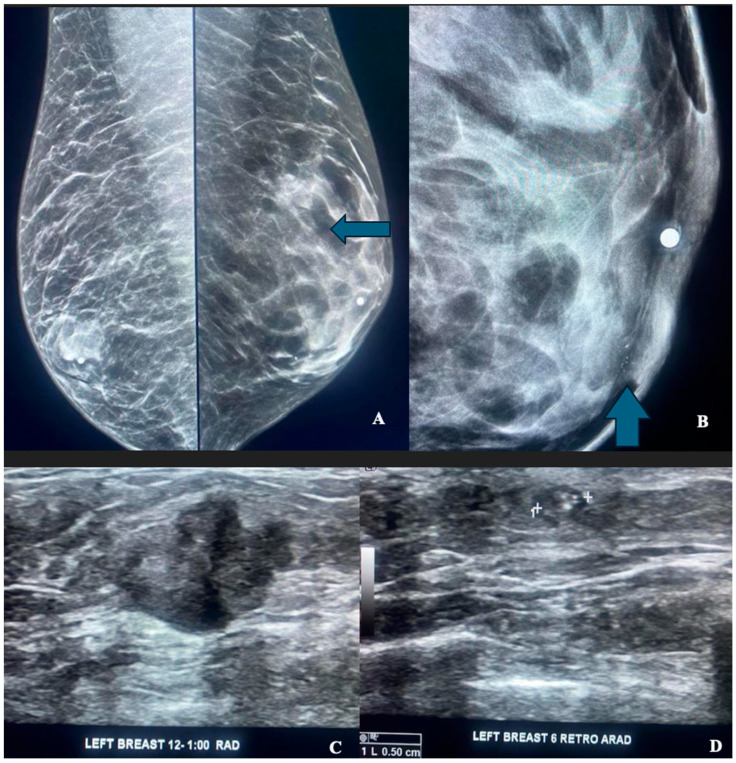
(A–D) Multifocal breast cancer of the left breast in a patient presenting with a palpable lump in the left breast. (A) Bilateral MLO view demonstrates bilateral gynecomastia, left greater than right, and a partially obscured mass (arrow) in the upper left breast within a background of dense breast tissue. (B) Calcifications at the left 6:00 axis anteriorly (arrow) are seen to better advantage on this magnification view. (C) Ultrasound delineates a suspicious hypoechoic mass at the left 12:00 to 1:00 axis, corresponding to a partially obscured mass in this region on mammography. Ultrasound-guided biopsy revealed invasive ductal carcinoma. (D) Ultrasound demonstrates an ovoid hypoechoic 5 mm nodule containing calcifications, corresponding to suspicious calcifications at the anterior left 6:00 axis on mammography. Ultrasound-guided biopsy revealed DCIS.
